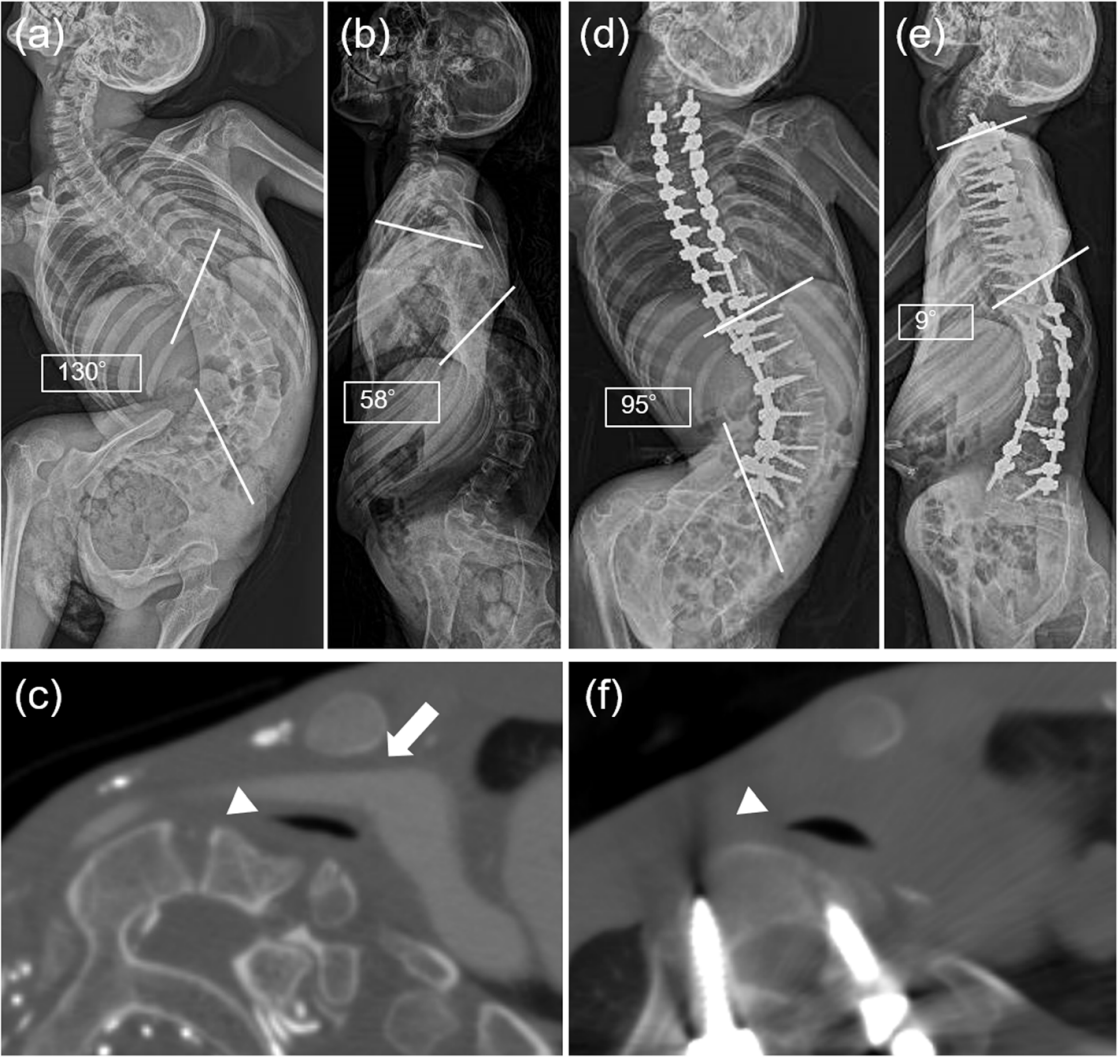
Image findings of case 2. a, b Preoperative anterior-posterior and lateral X-rays of the whole spine in supine position shows severe scoliosis with exaggerated cervicothoracic hyperlordosis. c Preoperative contrast enhanced computed tomography at T3/4 level demonstrates flattened tracheal lumen between innominate artery and anteriorly deviated vertebra. d, e Postoperative anterior-posterior and lateral X-rays of the whole spine in supine position reveals well-corrected cervicothoracic alignment. f Postoperative computed tomography demonstrates the improvement of tracheal stenosis. The white arrow in (c) indicates the innominate artery compressing the trachea from the anterior aspect. The white arrowheads in (c) and (f) indicate the tracheal lumen
